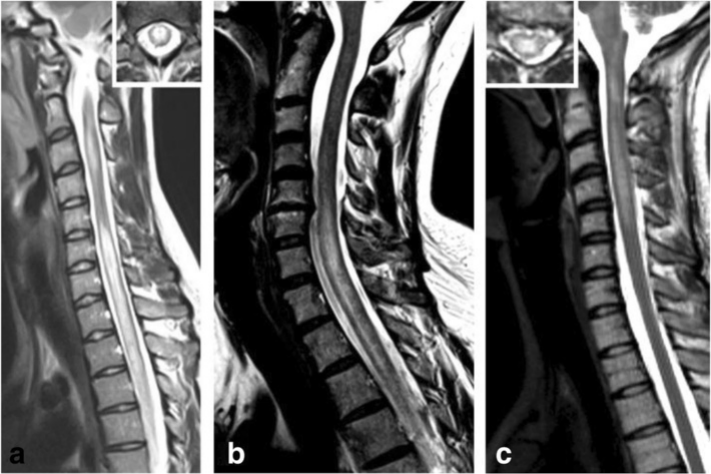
Examples of spinal cord MRI findings. a Sagittal T2-weighted spinal MRI performed at disease onset revealed a large longitudinal centrally located lesion extending over the entire spinal cord as well as swelling of the cord. b Longitudinal extensive central spinal cord T2 lesion in another patient. c T2-hyperintense lesions extending from the pontomedullary junction throughout the cervical cord to C5 in a third patient. The *insets* in A and C show axial sections of the thoracic cord at lesion level
